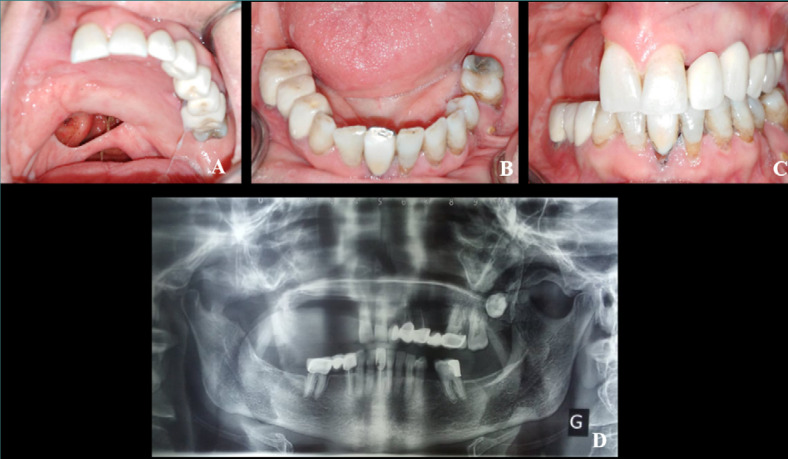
Initial situation. A–C, Intra oral views of the maxillary arch, velo-palatal defect, and mandibular arch. D, Panoramic X-ray.
